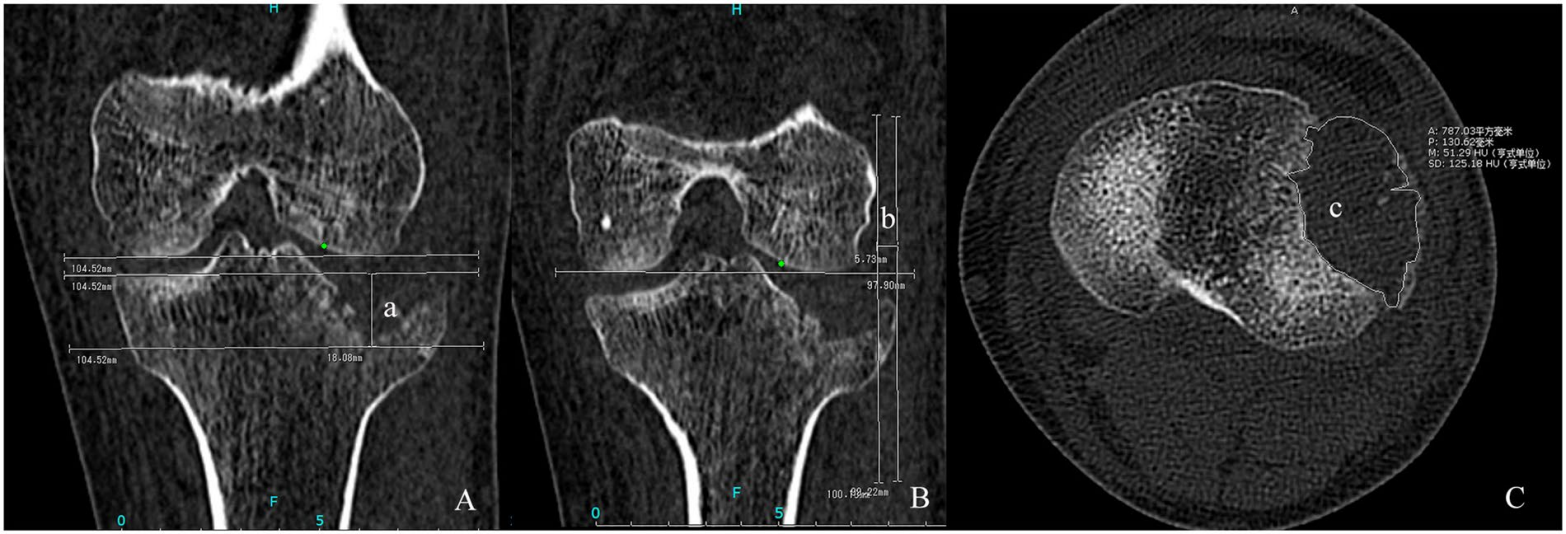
A 42-year-old male who sustained a type II fracture of tibial plateau. (A) Coronal reformatted CT image, a = the depth of lateral plateau depression, 18.08 mm; (B) Coronal reformatted CT image, b = lateral plateau widening, 5.73 mm; (C) Axial view of CT image, c = the area of lateral depression, 787.03 mm^2^.
